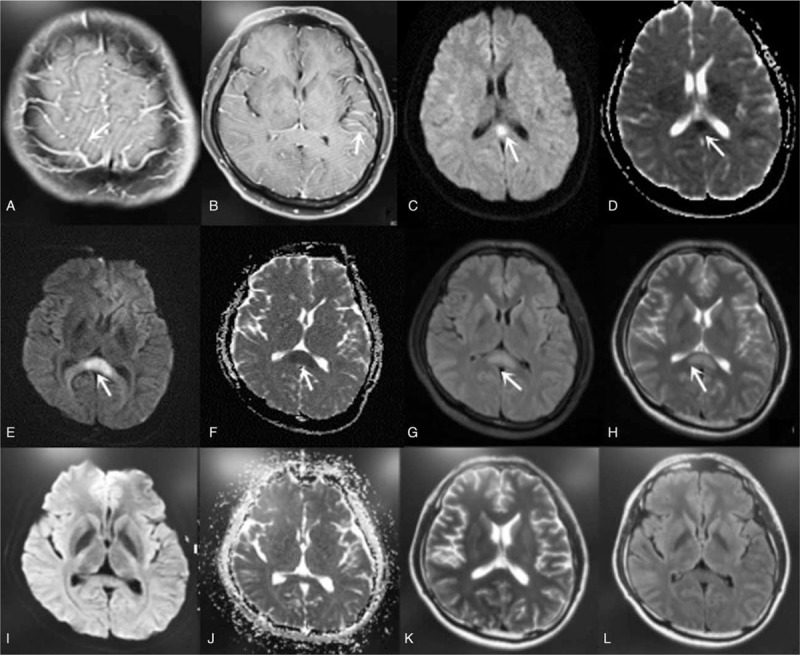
MRI+C (A, B) showed the blood vessels on the surface of the brain are obviously increased and strengthened. On day 4, DWI (C) showed high signal intensity and ADC (D) low signal intensity in the splenium of corpus callosum. On the 5th day, MRI showed FLAIR (G) and T2 (H) high signal in the splenium of corpus callosum, DWI (E) showed splenial hyperintensity as a “boomerang sign” and reduced diffusion on ADC (F) maps. Cranial MRI returned to normal on day 30 (I–L). ADC = apparent diffusion coefficient; MRI = magnetic resonance imaging.
